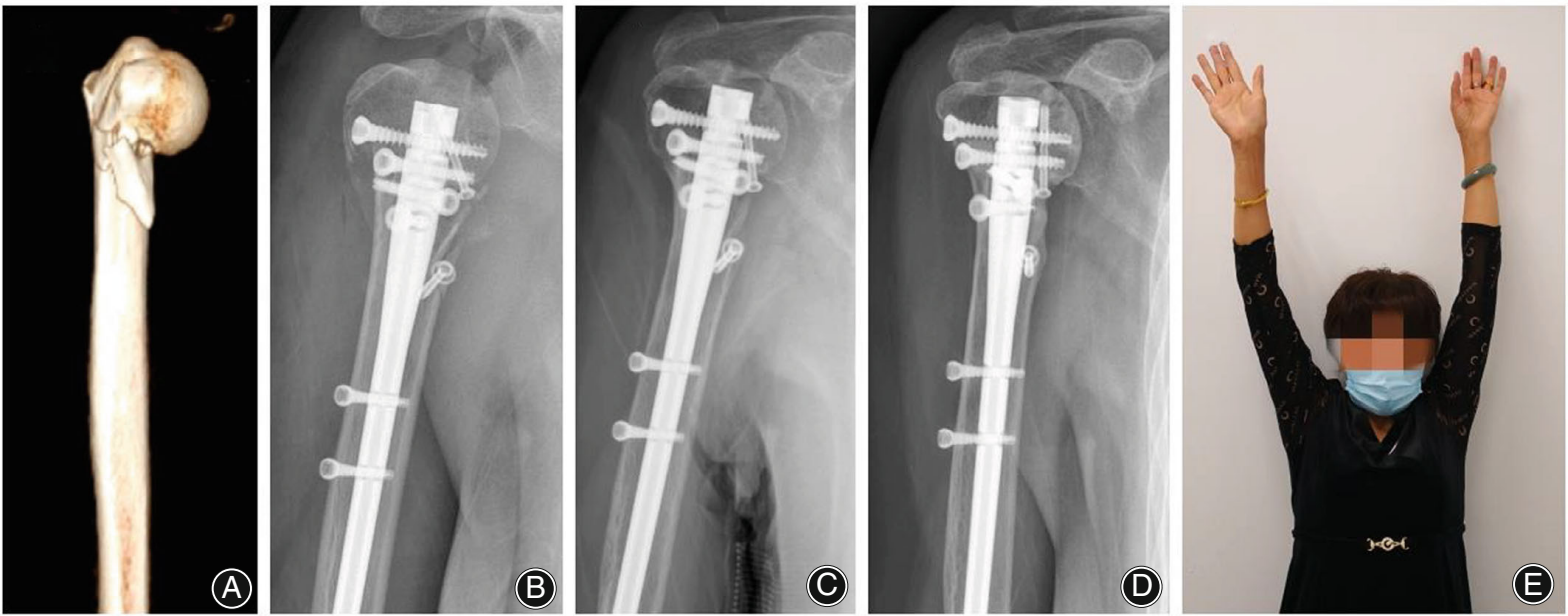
(A) Preoperative 3D‐CT; (B) Radiograph immediate postoperatively; (C) Radiograph 5 months postoperatively; (D) Radiograph 1 year postoperatively; (E) Functional image at final follow‐up
